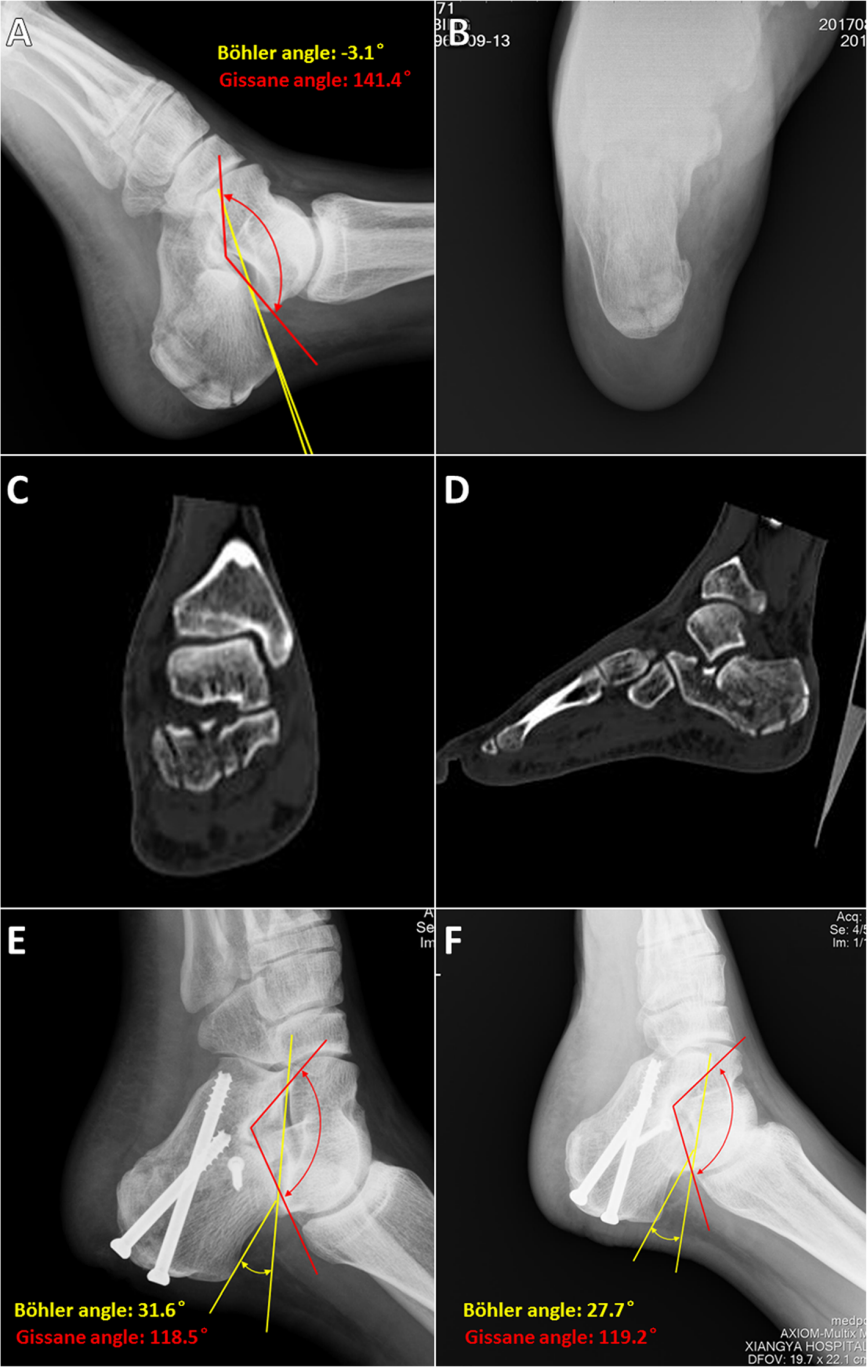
Imaging examination and evaluation of a representative case (male, 47 years old, left Sanders type IV fresh calcaneal fracture). a Example of an intra-articular fracture of the calcaneus. Böhler angle was − 3.1° and Gissane angle was 141.4°. b Axial view showing a varus deformity of the calcaneus. c, d Coronal and sagittal views of the calcaneus in the CT scan show an irregular facet of the subtalar joint (Sanders type IV calcaneus fracture). e Lateral radiograph obtained 2 days after the surgery confirmed anatomic reduction of the calcaneus and stable fixation. Böhler angle was 31.6° and Gissane angle was 118.5°. f Lateral radiograph obtained at approximately 12 months confirmed anatomic reduction and stable fixation, with a Böhler angle of 27.7° and Gissane angle of 119.2°
